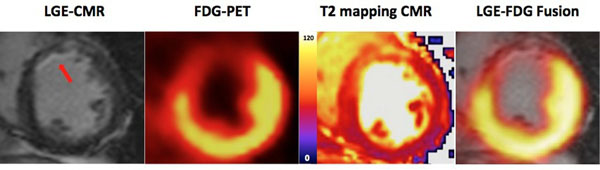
Multi-modality imaging of Subendocardial MI (arrows) with significant myocardial salvage. The area of reduced myocardial FDG uptake and edema on T2 mapping MR imaging (the AAR) extends transmurally and radially beyond the infarcted area (LGE, MR).
